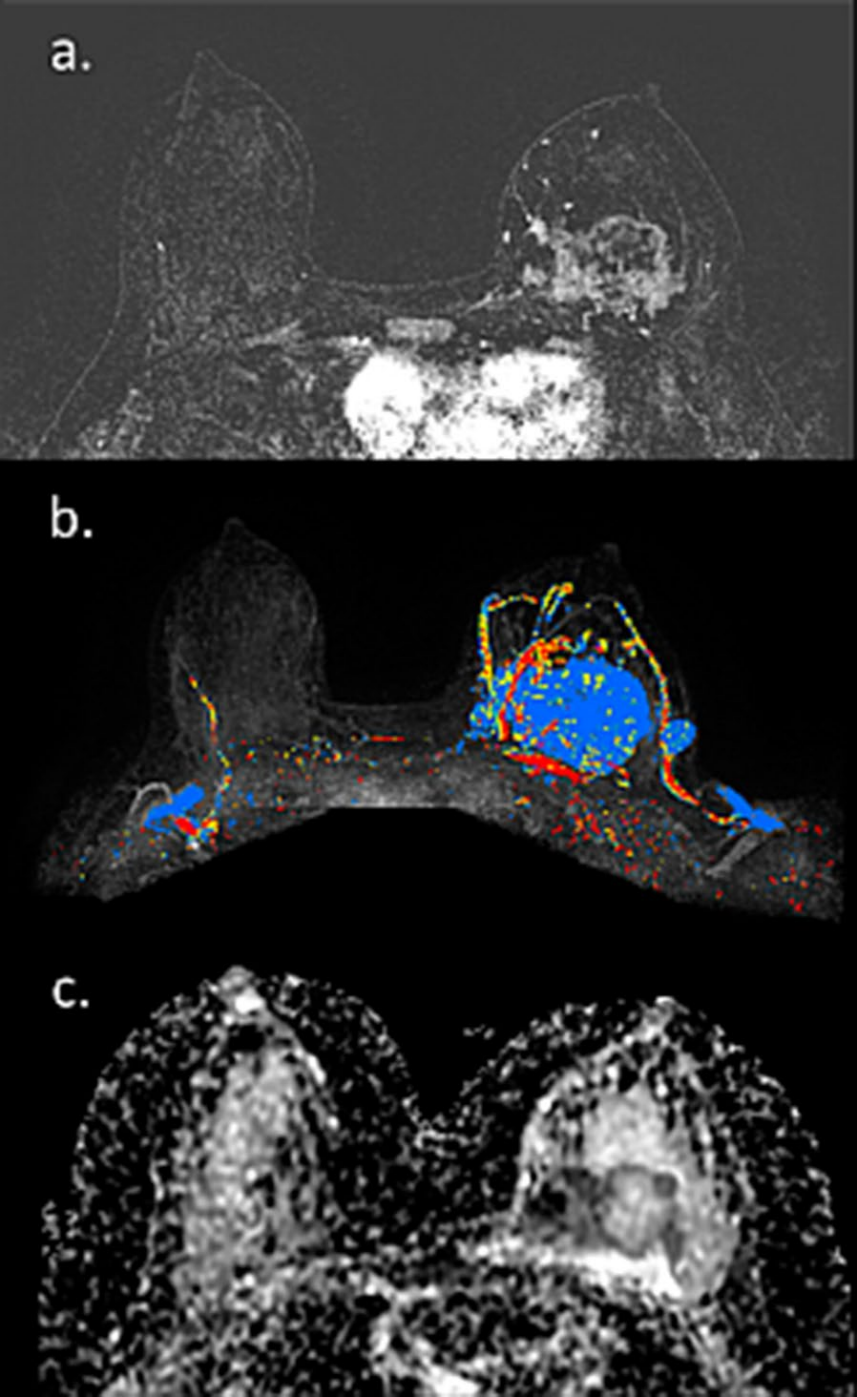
MRI of delayed diagnosed IDC during lactation. Axial subtraction DCE-MRI, CAD and ADC map of a 25-year-old PABC patient lactating for 8 months, with a 6.7 cm triple negative IDC on the right breast are presented. The massive lesion exhibited with an irregular rim enhancement concordant with triple negative IDC and is clearly visible on top of the surrounding mild BPE (a), with mostly persistent enhancement kinetic pattern (b) and decreased ADC values in margins of the lesions, with increased diffusivity in the central necrotic region (c)
